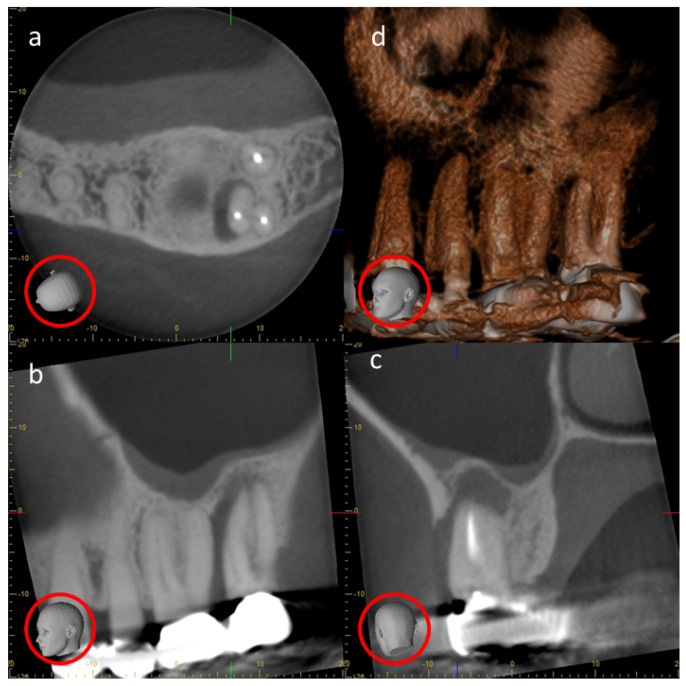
Depiction of a 4 cm × 4 cm field-of-view (FOV) cone-beam computed tomography (CBCT) of a periapical–periodontal lesion on an already-root-treated molar tooth. This is an orthogonal, multiplanar reconstruction: (a) is axial, (b) is sagittal and (c) is coronal. The ‘heads’ indicated by the red circles clarify their orientation. (d) is a 3D reconstruction of the whole 4 × 4 dataset. (a–c) are Adapted from Figure 3, I Reference [6] MacDonald, D.: Reitzik, S. Int Dent J 2022, 72, 448-55.
